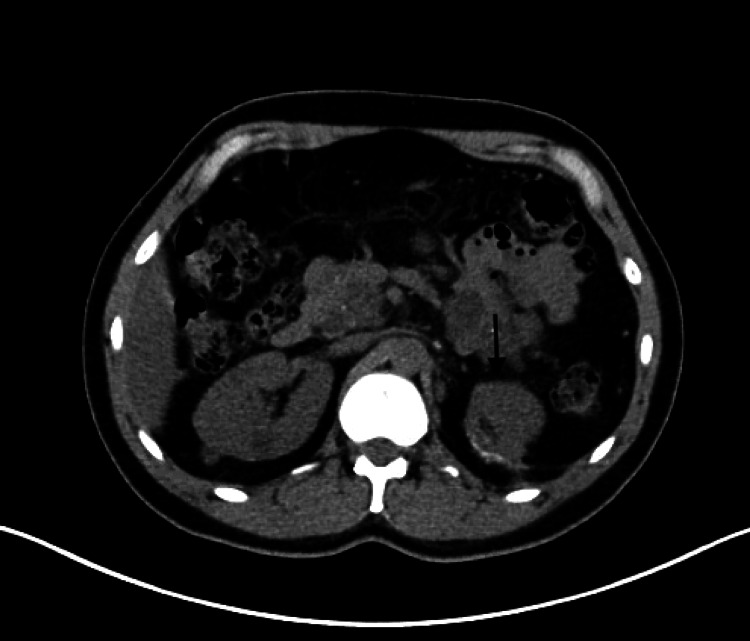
CT abdomen (three months post partial nephrectomy) revealed no tumor recurrence CT: computed tomography
